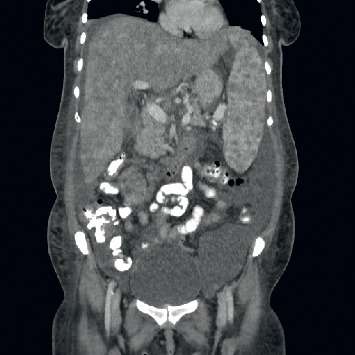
Computed tomography of the pelvis with contrast showing densities in the spleen.
